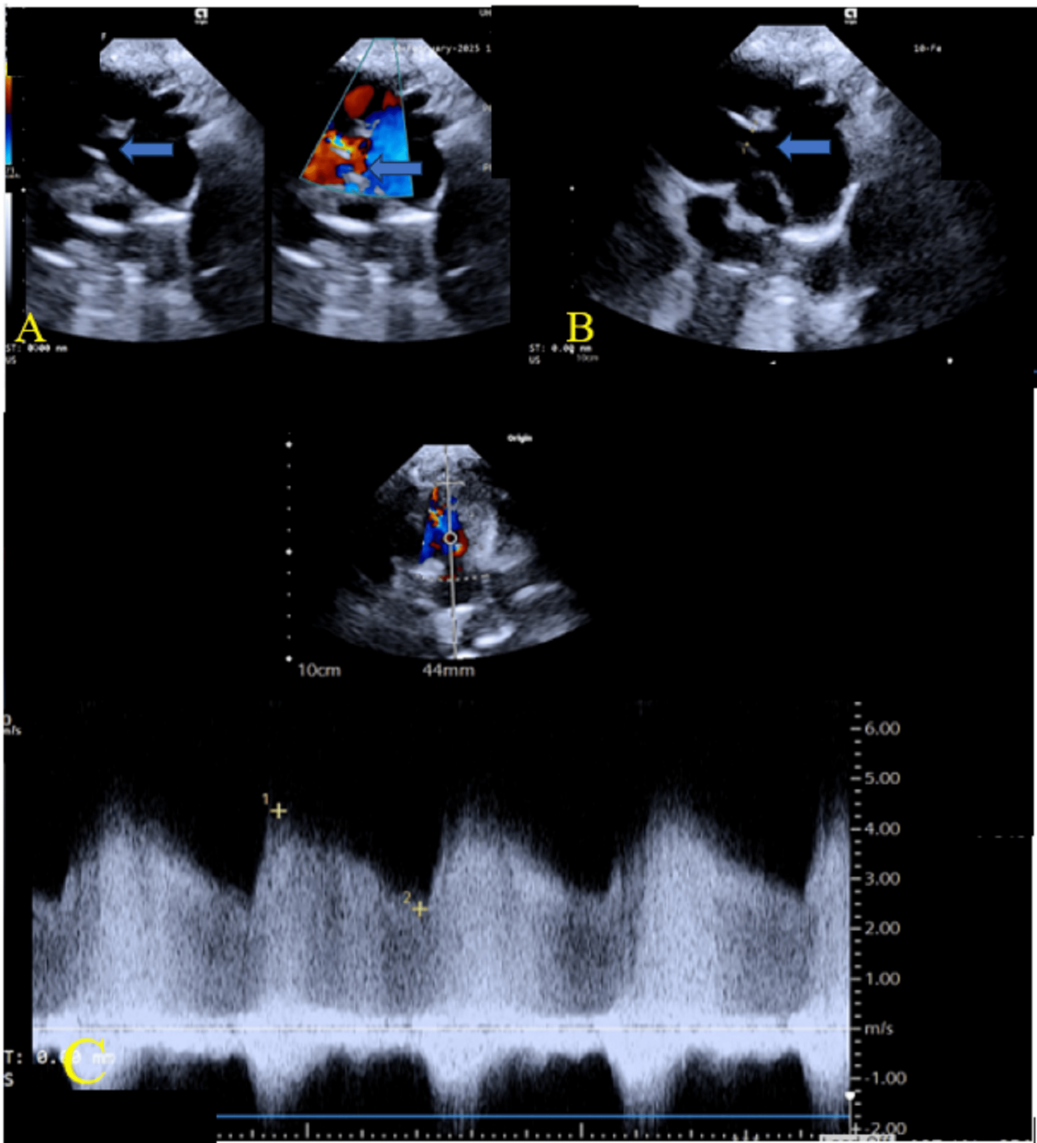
Transthoracic echocardiogram demonstrating a moderately sized short conical 5mm patent ductus arteriosus (PDA) with a left-to-right shunt. A: 2D and color Doppler (arrow). B: PDA measuring 5mm (arrow). C: Continuous-wave (CW) Doppler showing continuous flow.
